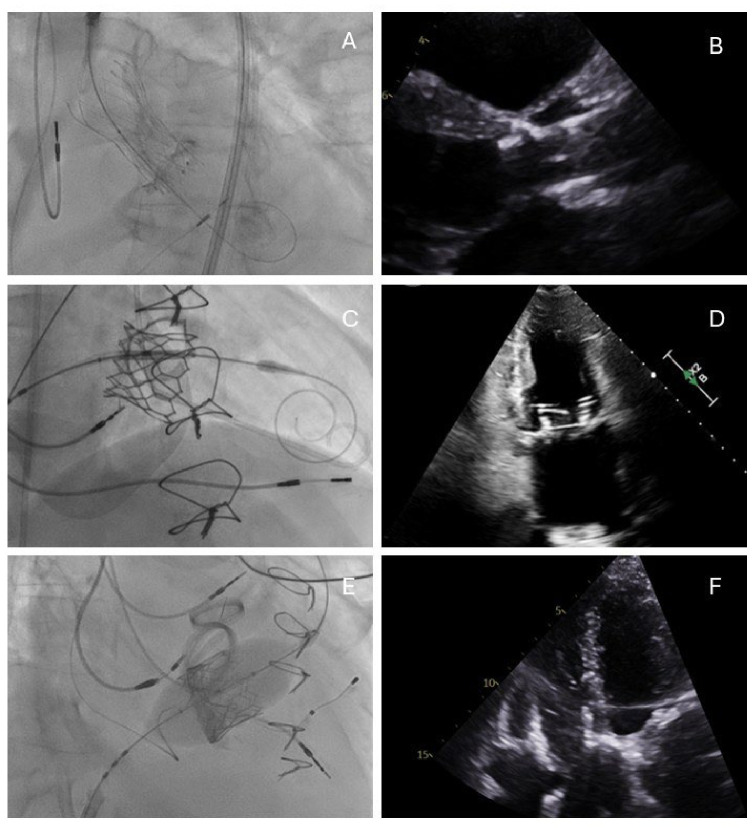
Valve-in-valve (ViV) TAVI, shown on both fluoroscopy and transthoracic echocardiography. Panels (A,B) show ViV in the aortic position with a self-expanding valve, with the left ventricular delivery wire loop still at the apex. Panels (C,D) show ViV in the mitral position with a balloon-expandable valve, with a pigtail catheter delivered through the aortic valve to left ventricular apex for invasive haemodynamic assessment. Panels (E,F) show ViV in the tricuspid position during balloon inflation for valve deployment. Note the mechanical mitral and aortic valve replacements in the background.
